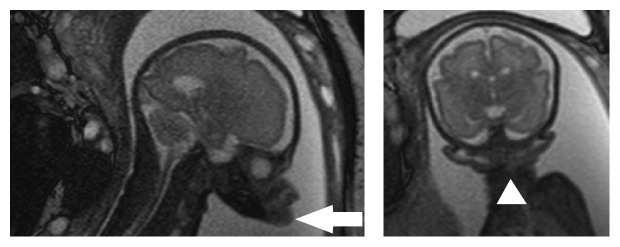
Magnetic resonance imaging demonstrated agnathia with low-set ventromedial displaced ear (arrowhead) and small opening of the mouth (arrow).
